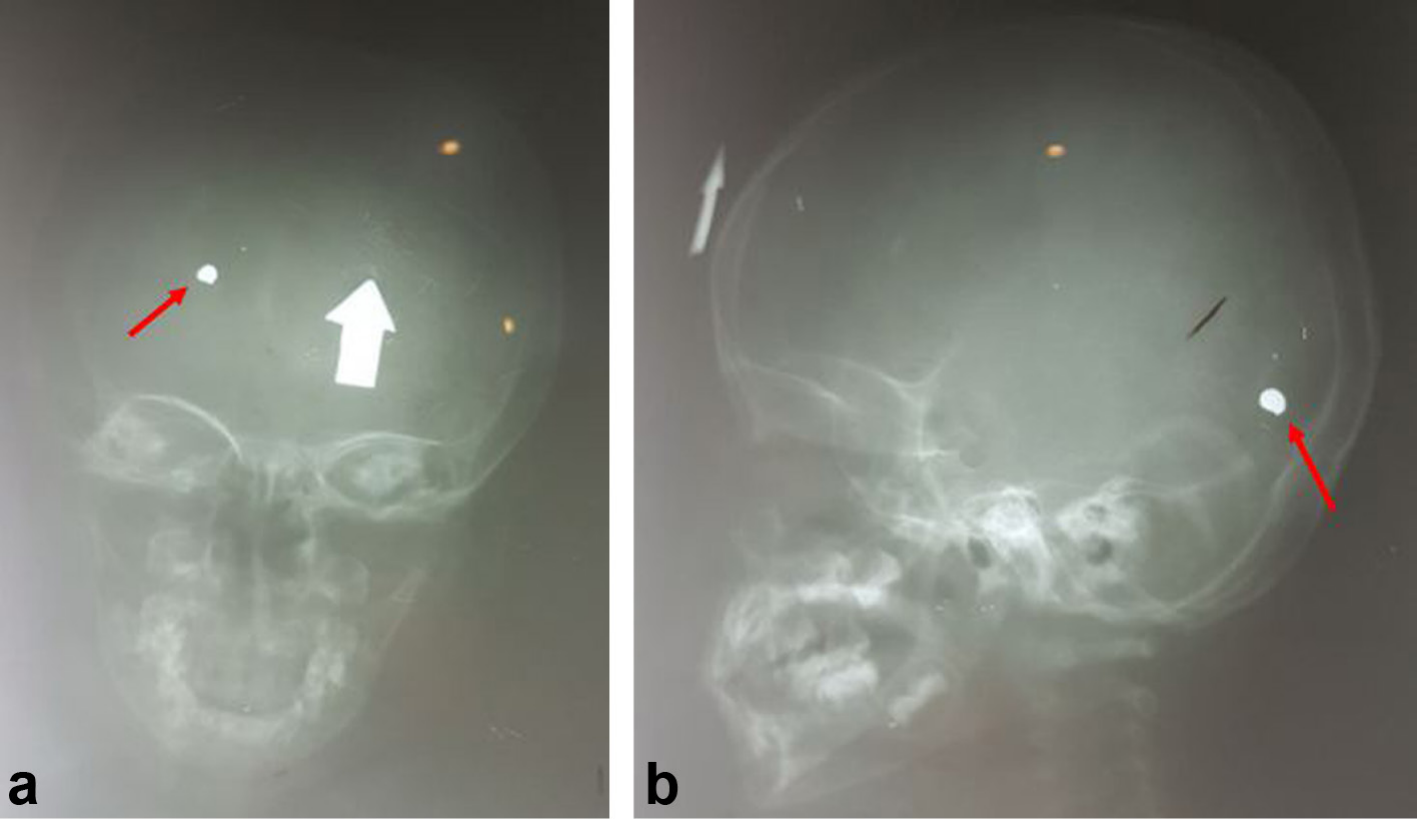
Skull radiograph anteroposterior/lateral (a, b). A metal-opacity foreign body (red arrow) is detected at the right occipital lobe with fracture at the left frontal bone. A marker must be used in radiography to mark the entry wound (white arrow).
